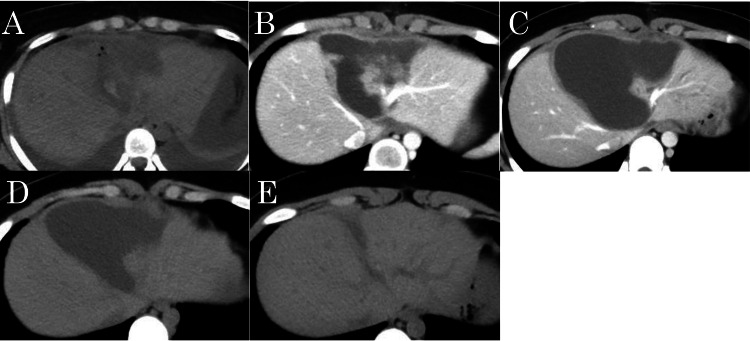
Computed tomography after hepatorrhaphy demonstrating changes over time (A) 7 days; (B) 21 days; (C) 2 months; (D) 3 months; (E) 5 months.
